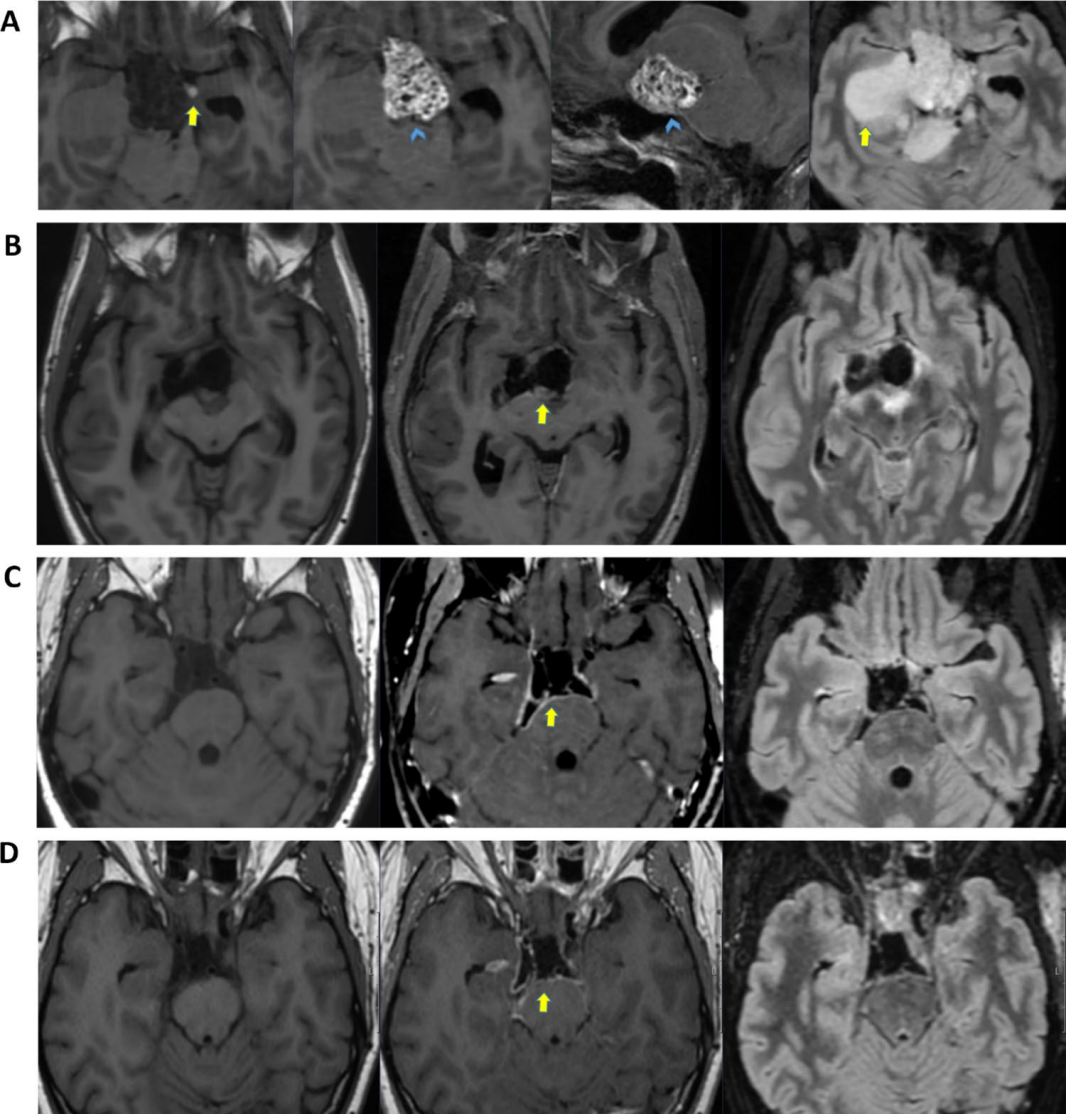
A) Axial native T1-w, CE T1-w, sagittal CE T1-w, and axial T2-FLAIR. A centrally located tumor with both calcified and cystic non-contrast-enhancing components (yellow arrow) and contrast-enhancing components (blue arrow-head), causing obstructive hydrocephalus. Craniopharyngioma was given as the top diagnostic differential. B) Axial native T1-w, CE T1-w, and axial T2-FLAIR. Good postoperative status with suspicion of only minimal residual tumor in the interpeduncular cistern (yellow arrow). C) Axial native T1-w, CE T1-w, and axial T2-FLAIR. Extra-axial contrast-enhancement along the resection margins was seen, of difficult interpretation if related to residual tumor-related changes or meningitis-related changes. Renewed MRI after meningitis treatment completion is recommended in the radiological report. D) Axial native T1-w, CE T1-w, and axial T2-FLAIR. Unchanged extra-axial contrast-enhancement compared to the previous MRI exam, no signs of restricted diffusion, thus no radiological suspicion of meningitis. Still, of difficult assessment whether the extra-axial contrast-enhancement is related to postoperative changes or minimal tumor recurrence/remnant tumor. Further follow-up with an MRI in 3–6 months is suggested in the radiological report for reassessment.
